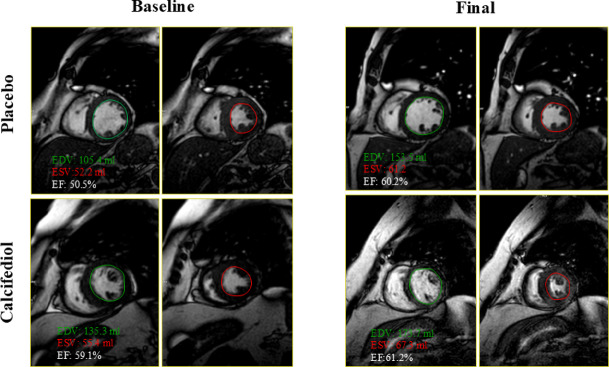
Representative examples of baseline and final cardiac magnetic resonance imaging (MRI) in a patient on placebo (number 33) and another treated with calcifediol (number 19). Green lines mark the left ventricular end-diastolic volume (EDV) and red lines correspond to the end-systolic volume (ESV). EF: Ejection fraction.
